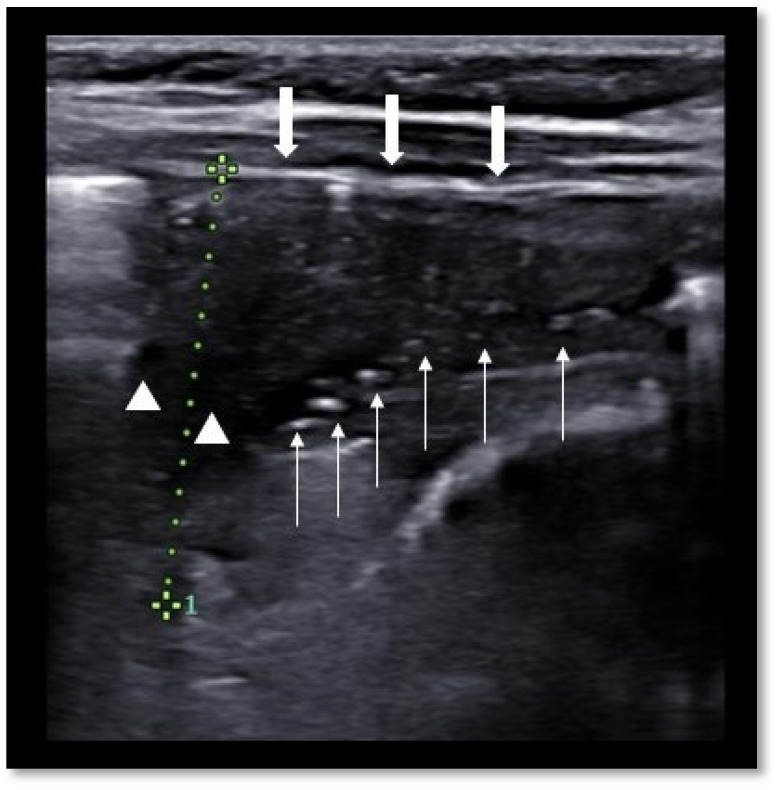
Basal lung consolidation in a 8 month-old girl, showing hypoechoic triangular shape, pleural line attenuation (thick arrows), air (thin arrows) and fluid (triangles) bronchograms. Depth is approximately 2.5 cm (dotted green line). Ultrasonographic appearance is compatible with pneumonia. Image captured using a 3.0–16.0 MHz linear array transducer.
